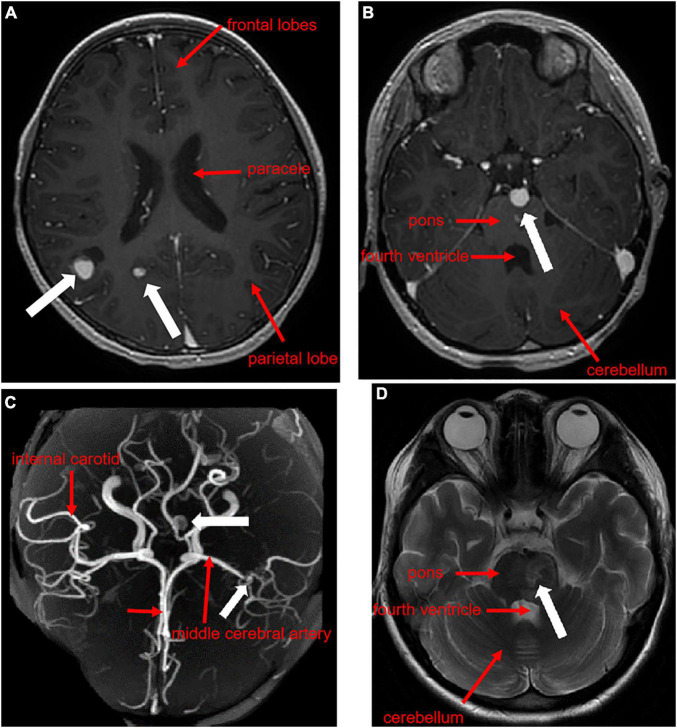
Contrast-enhanced cranial MRI + MRA of the pediatric patient. The transverse plane through the inferior portion of the parieto-occipital sulcus showed a distal aneurysm of the right middle temporal artery and the right posterior cerebral artery (A), the transverse plane through the superior cerebellar peduncle showed an aneurysm at the tip of the basilar artery and stenosis of the left middle cerebral artery with multiple collateral vessel formation (B,C), and the transverse plane through the superior cerebellar peduncle showed an abdominal cerebral infarction (D).
